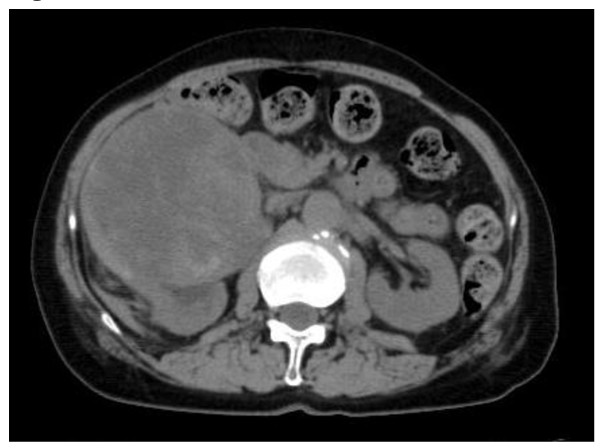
Abdominal CT showed right renal mass, suggestive of RCC at the first visit.
